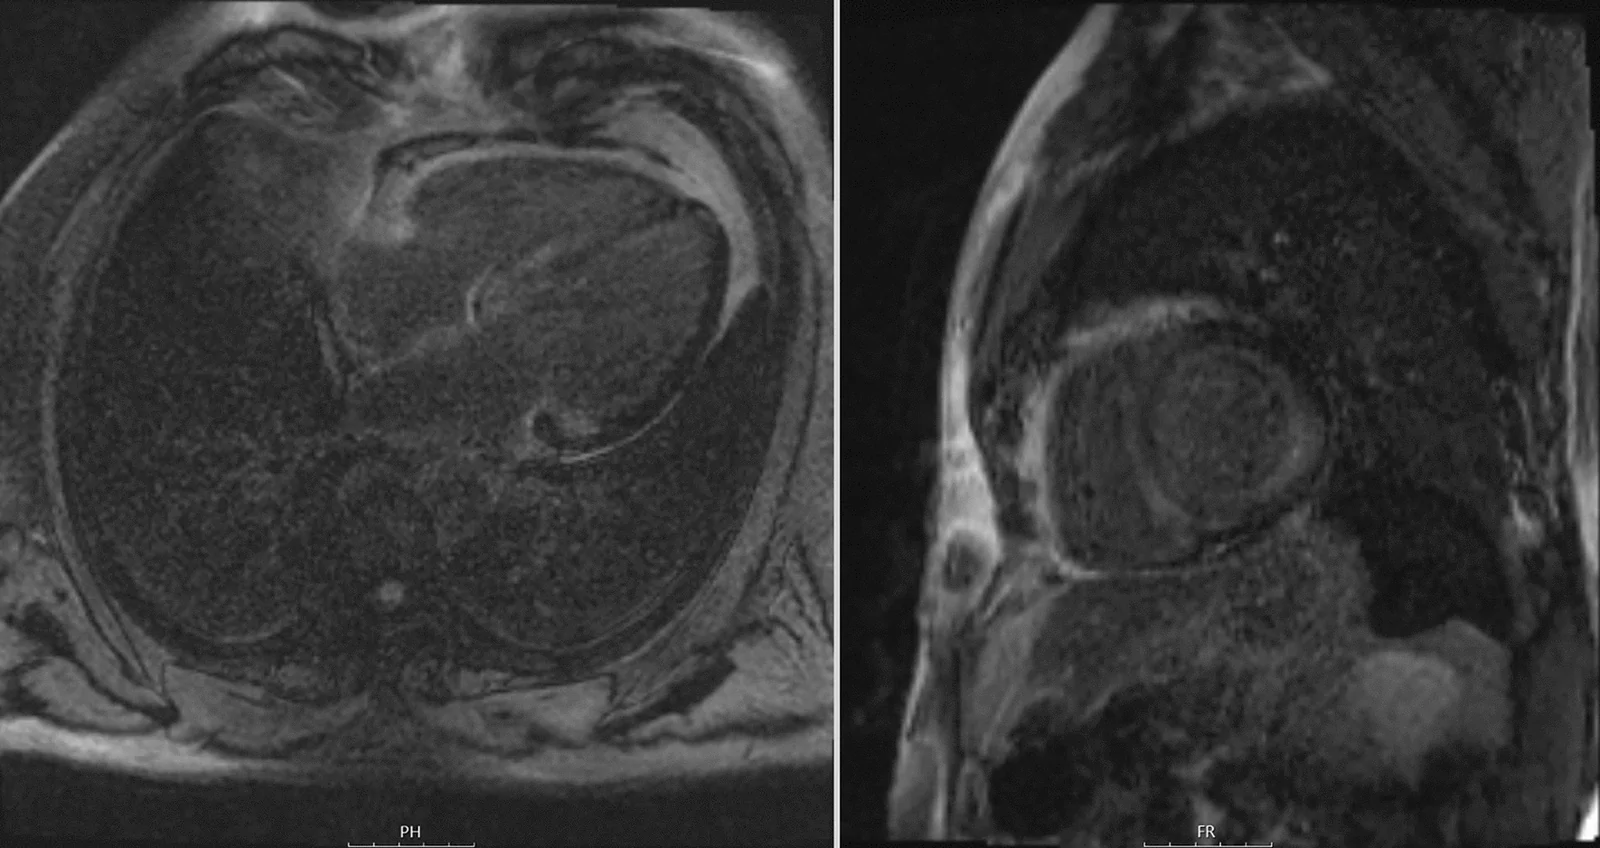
Cardiac magnetic resonance imaging: severe left ventricular hypertrophy (20 mm at the inferoseptal wall); dIffuse left ventricular fibrosis was seen, predominantly in the subendocardium, and more noticeable in the basal segments; late-gadolinium enhancement pattern suggesting infiltrative cardiomyopathy
